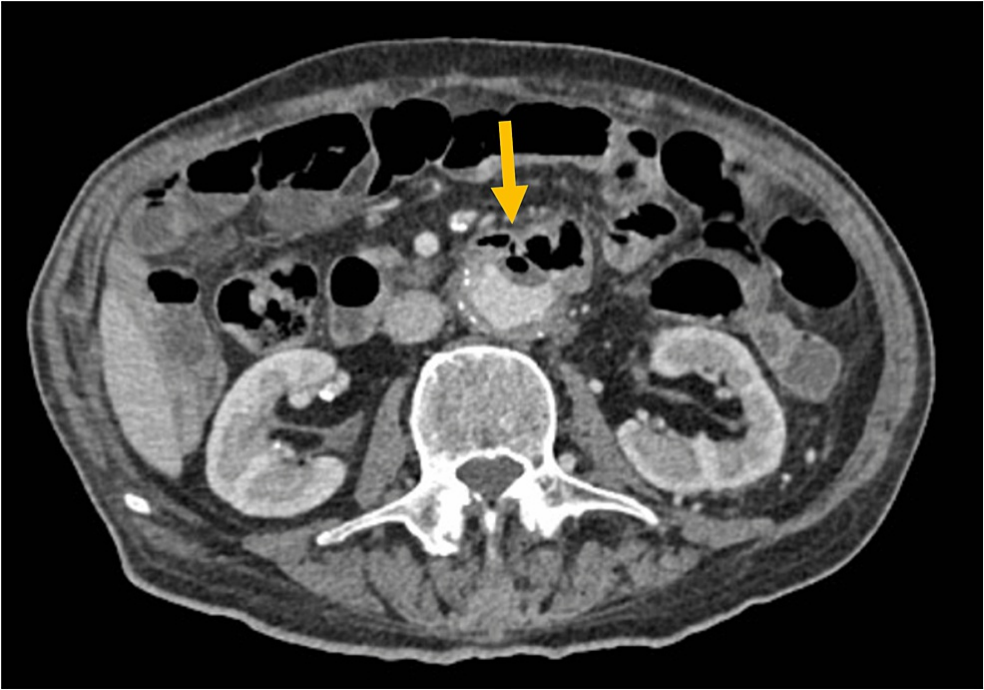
CT scan, infrarenal cut. Arrow pointing to gas in the aortic artery.
